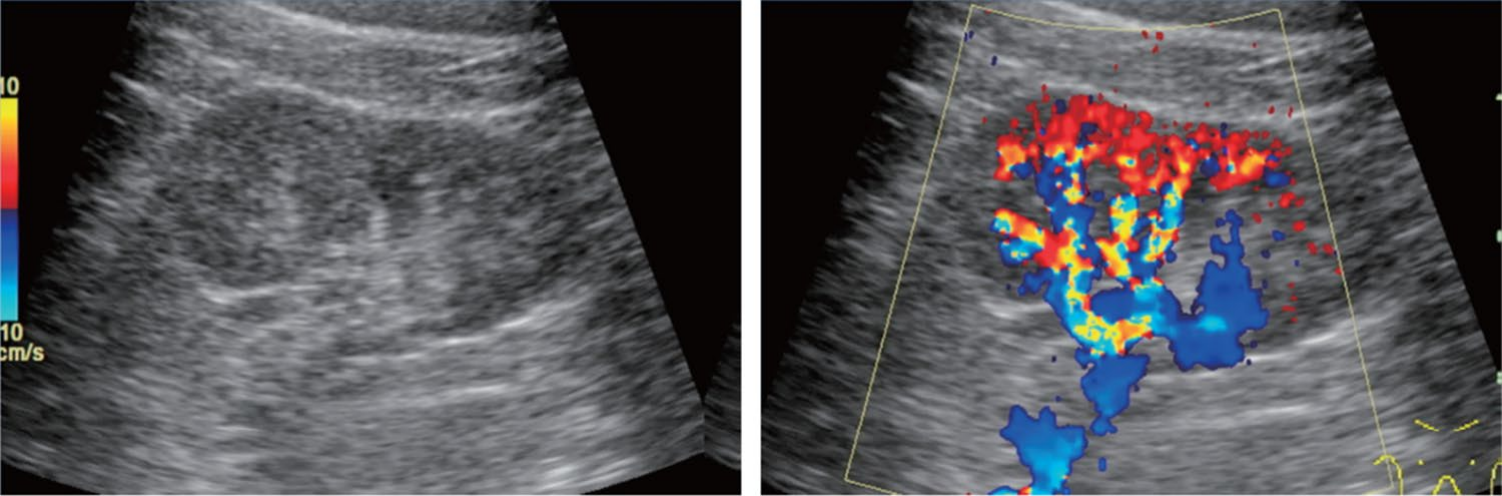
Morphological abnormality. A rugged contour is present (vascular structure similar to that of the normal renal parenchyma on color Doppler). Left: renal long-axis view; right: color Doppler. Category 2, Assessment B
